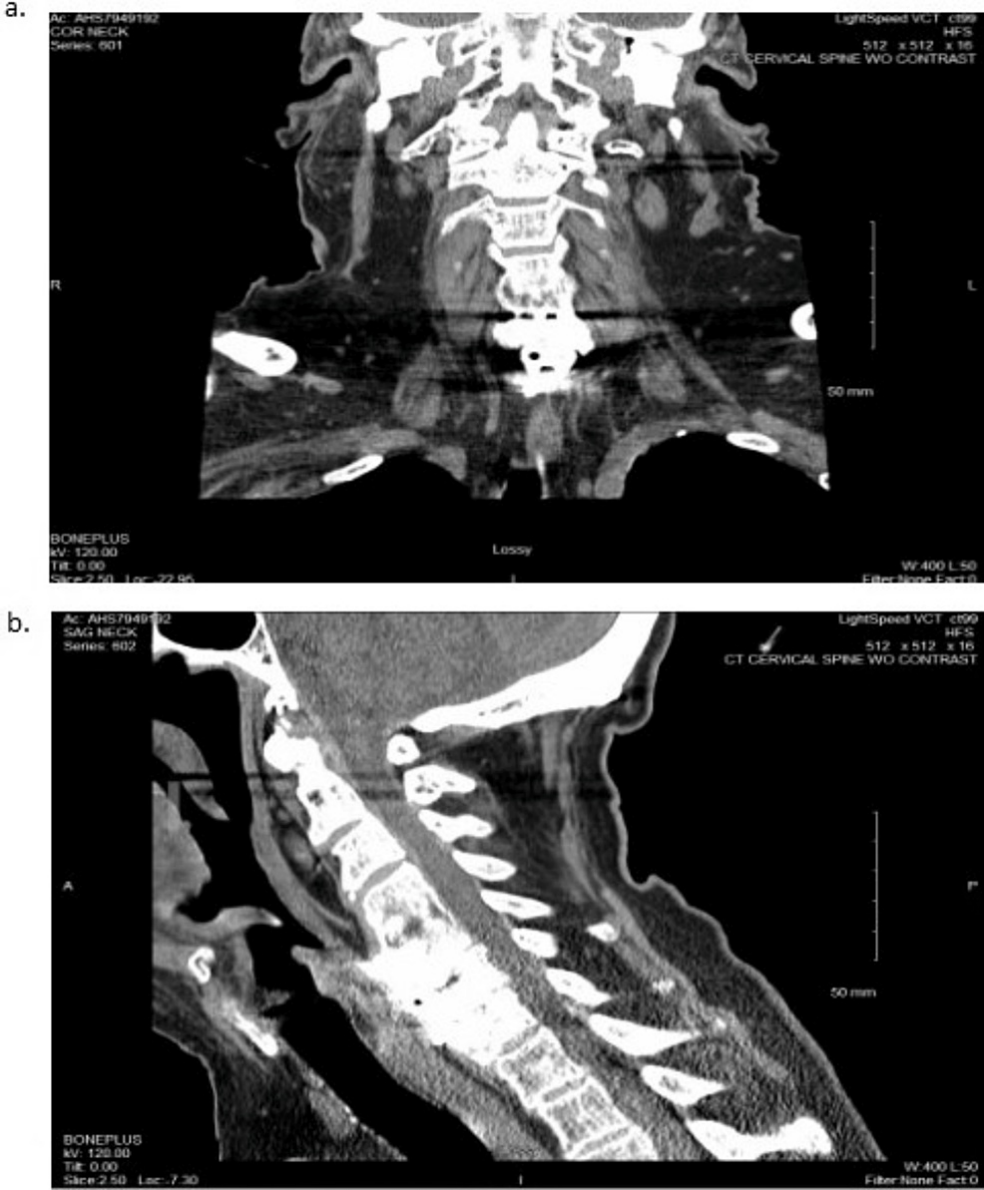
CT of the Cervical Spine Without Contrast a: coronal plane, b: sagittal plane CT: computed tomography
